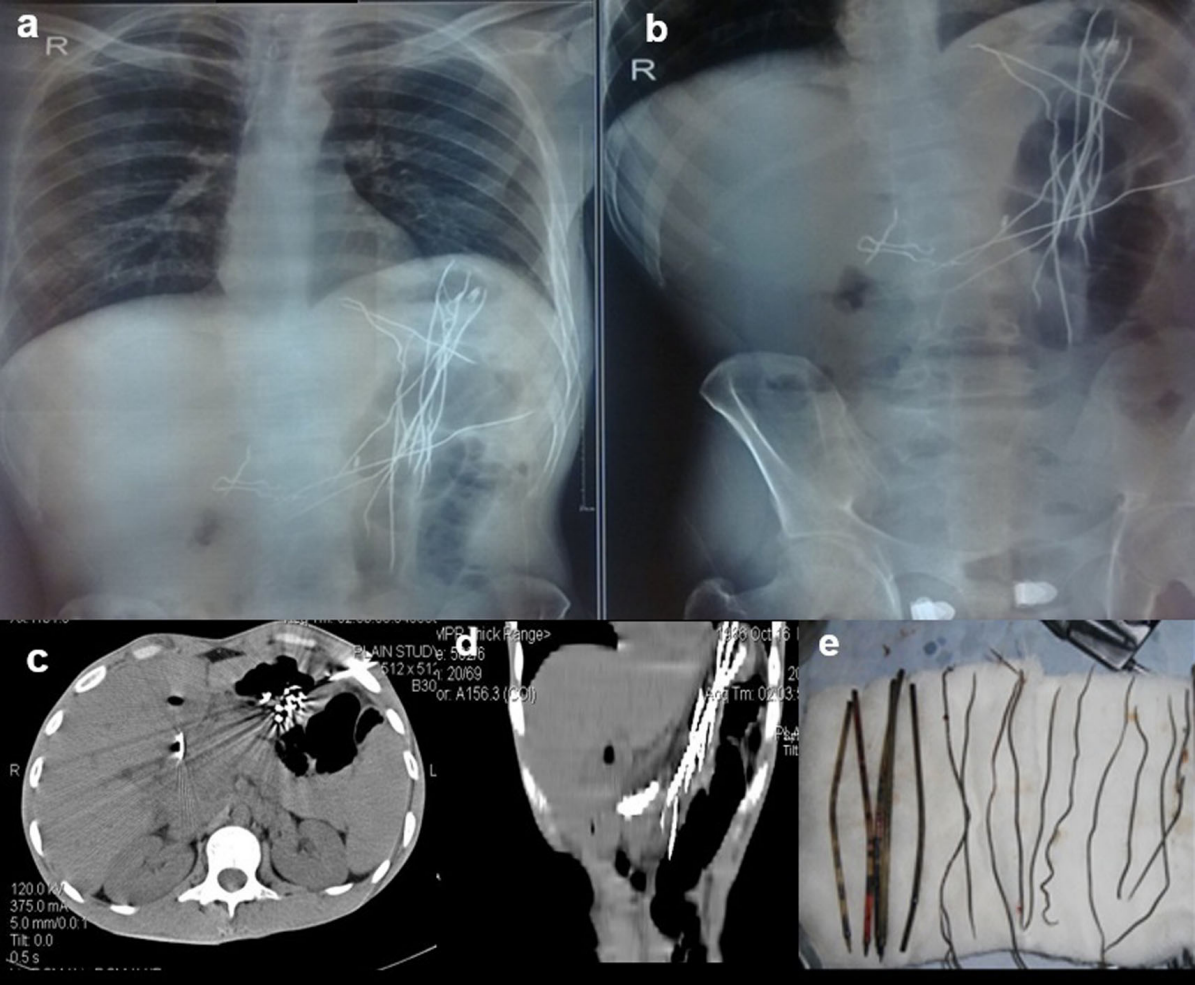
a-e: A Radiograph of the chest and abdomen reveal multiple linear radio-opacities in the left hypochondriac and lumbar quadrants of the abdomen (a & b), and a plain CT scan axial and sagittal image shows multiple hyperdense linear metallic foreign bodies within the gastric lumen, many piercing the gastric wall partially without any e/o perforation (c & d). On laparoscopy, multiple refills of the pen and wires were found in the stomach, which were removed (e).
